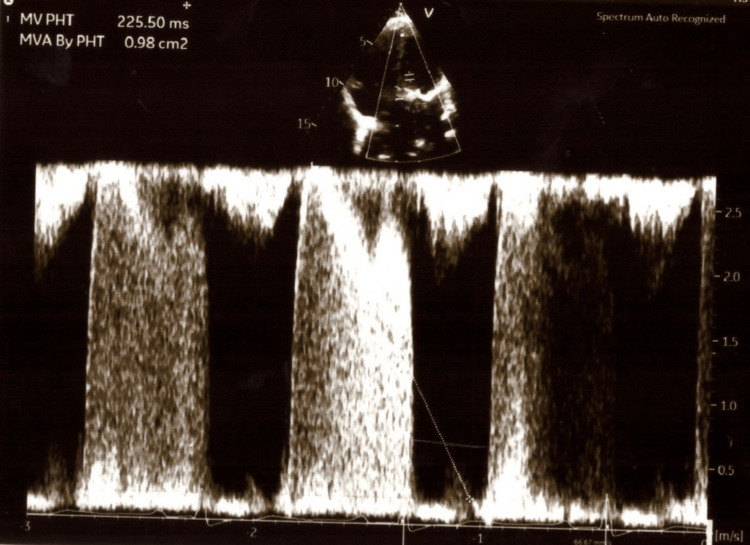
Transthoracic echocardiogram showing the mitral valve area
